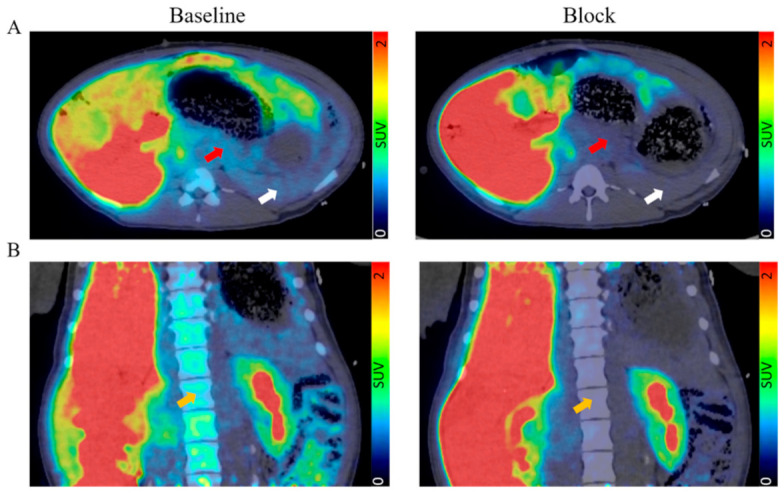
(A) Transversal and (B) coronal view of fused PET/CT scan with the averaged signal from 60–90 min. Red arrow indicates pancreas, white arrow indicates spleen, and orange arrow indicates bone marrow.
